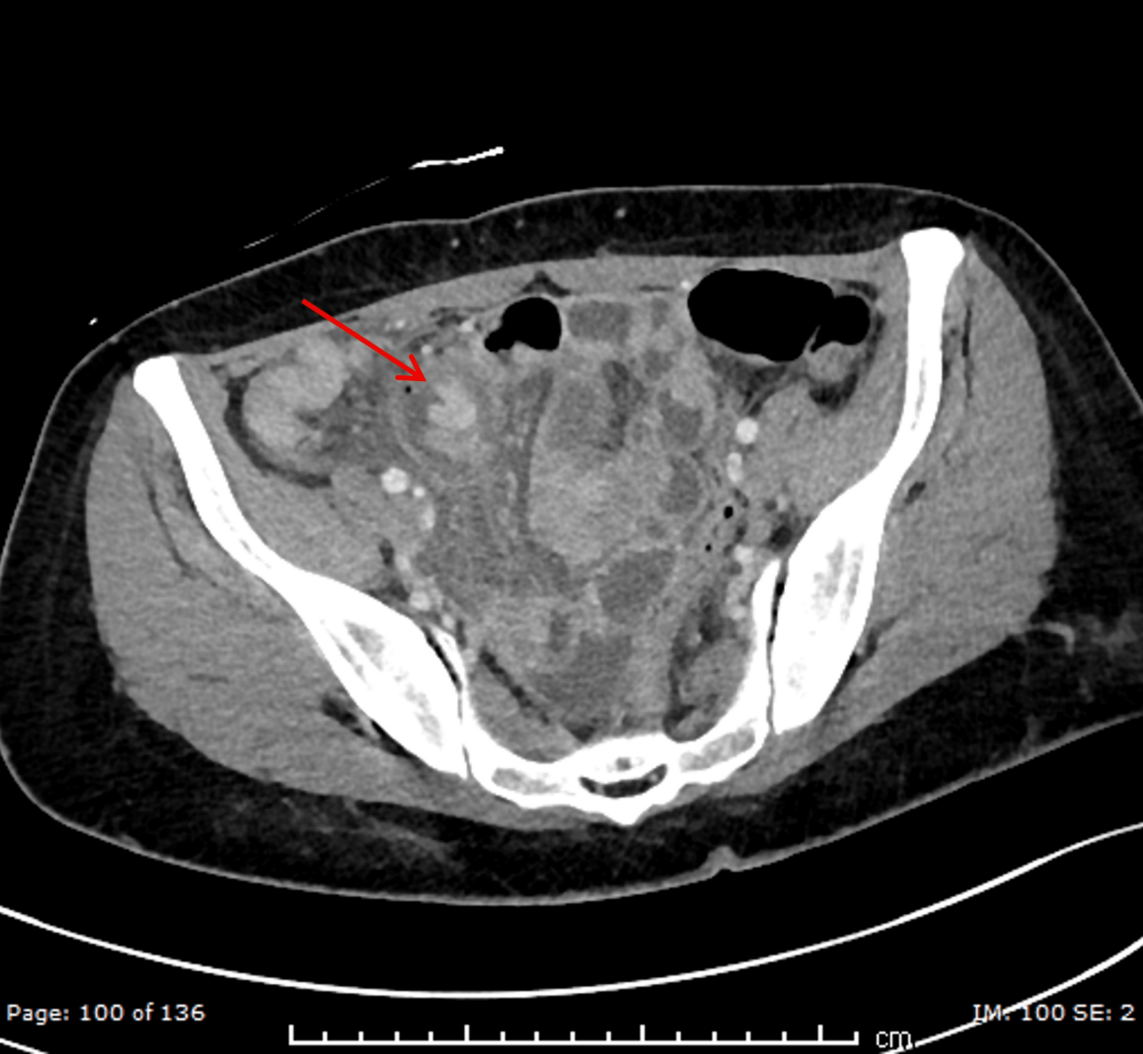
Axial section contrast-enhanced CT showing Meckel’s diverticulum, along with localized air nearby suggesting perforation. The red arrow is pointing to a thick-walled, blind-ending tubular structure arising from the distal ileum with focal wall interruption and adjacent fat stranding, consistent with perforated Meckel’s diverticulum.
